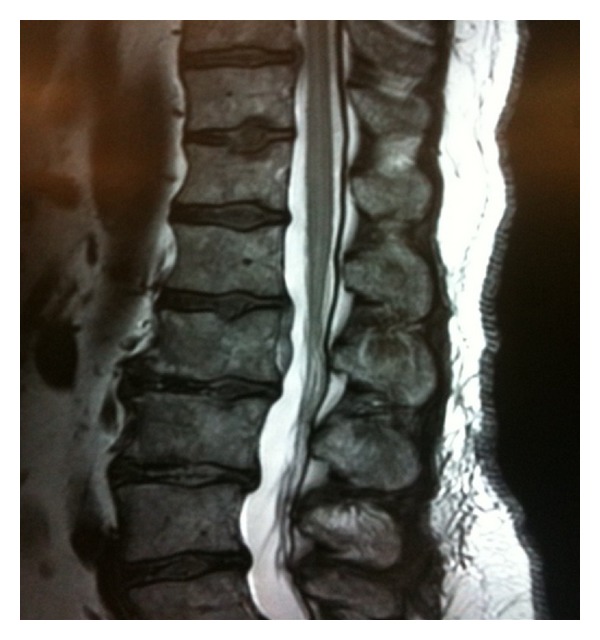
MRI of the lumbar spine showing acute spinal cord infarction. Sagittal T2-weighted MRI of the patient's lumbar spine showing a nonspecific central intrinsic signal hyperintensity of the spinal cord located in conus region with extension to T8 level. Image also shows multilevel degenerative disc disease with noncompressive disc herniation at L1-L2.
